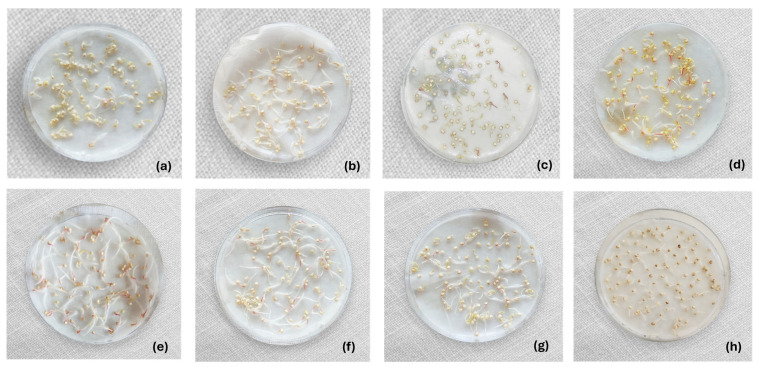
Visual comparison of Rosada de Huancayo (RH) quinoa grain germination after seven disinfection treatments: (a) 4% acetic acid; (b) 200 ppm sodium hypochlorite; (c) 70% alcohol (d) 8% hydrogen peroxide; (e) ultrasound 15 min + 200 ppm sodium hypochlorite; (f) ultrasound 30 min + 200 ppm sodium hypochlorite; (g) water 50 °C + 2% hydrogen peroxide + acetic acid 0.1%; (h) control.
